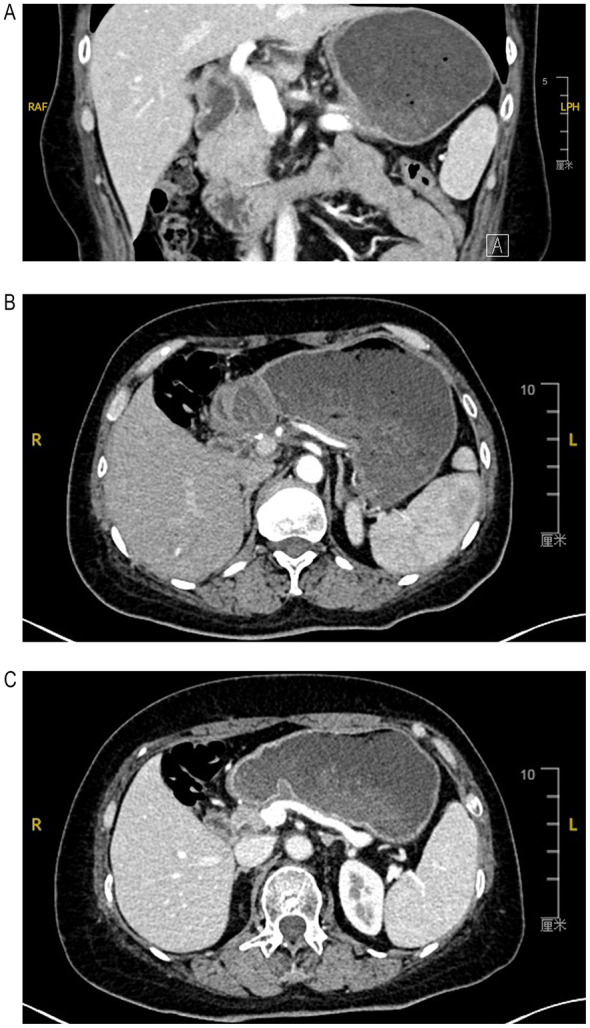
Surveillance imaging performed at 6 months postoperatively (CT). (A) The imaging confirmed complete tumor resection, with no evidence of local recurrence or distant metastasis. (B, C) The imaging demonstrated patent splenic artery and vein with well-maintained splenic perfusion.
